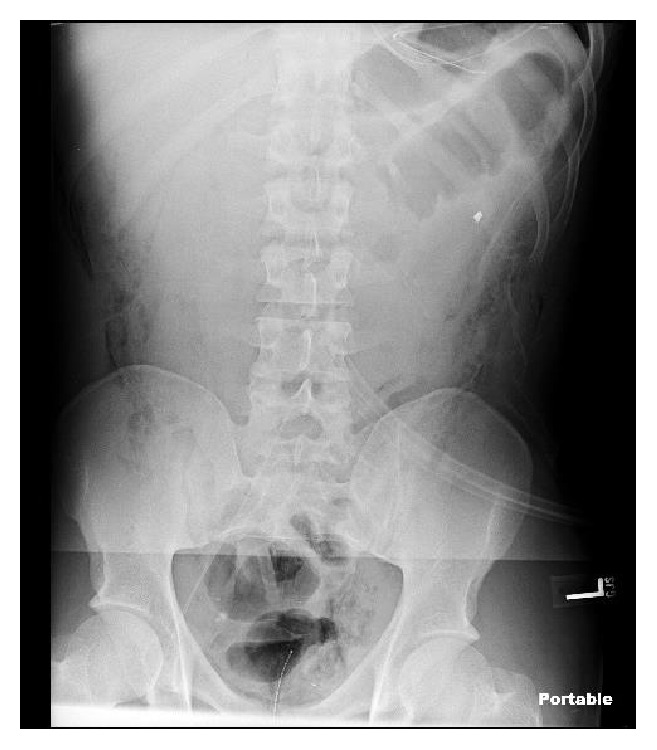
Post-op abdomen X-ray showing bullet fragment in left upper quadrant.
